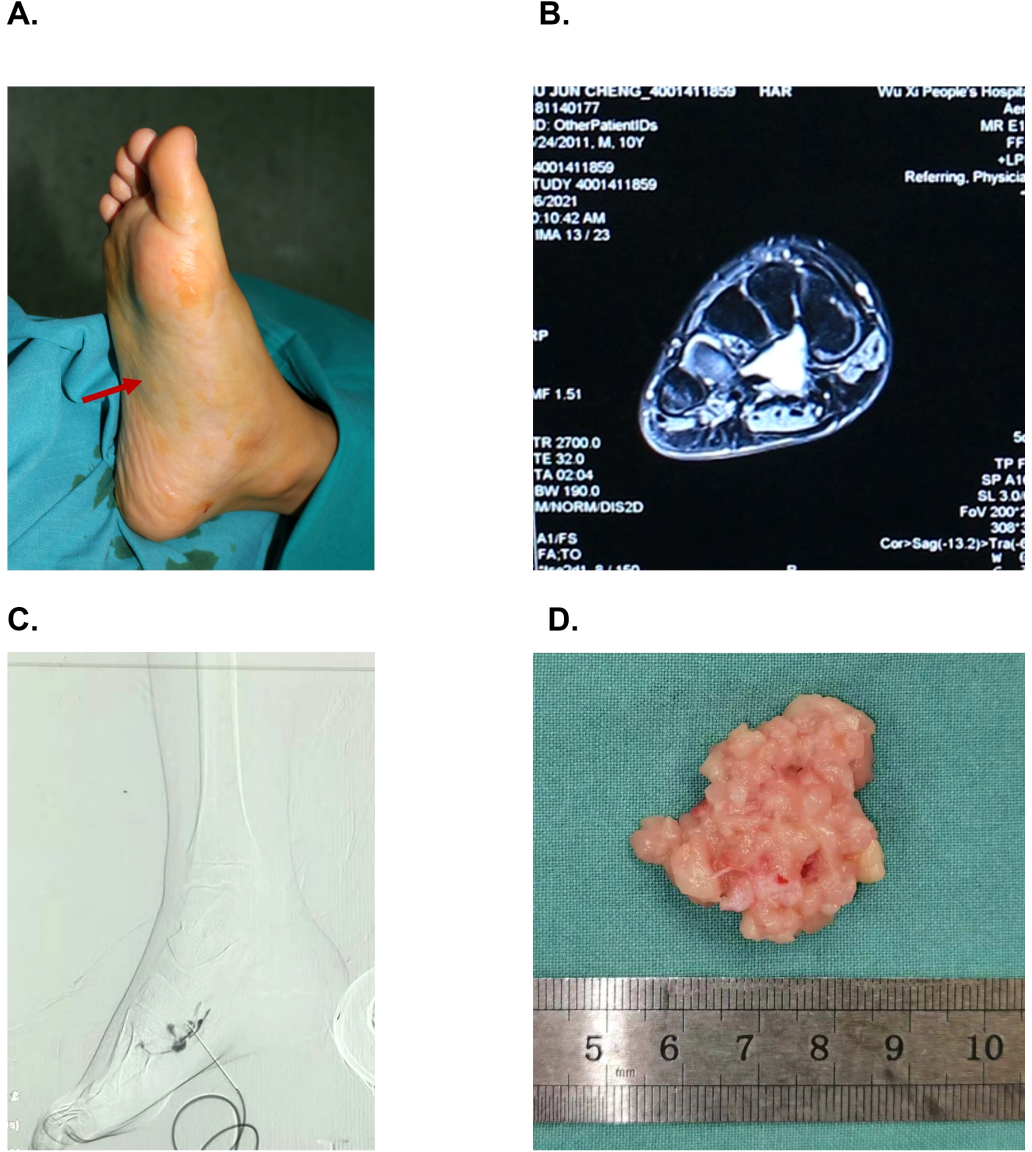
Patient diagnosis and management. The patient presented with a painful mass in right plantar (A). MRI revealed a high signal on T2-weighted enhanced sequence (B). The initial diagnosis was venous malformation, and sclerotherapy under DSA was performed, followed by surgical excision (C). Pathological biopsy of the lesion was subsequently established (D).
